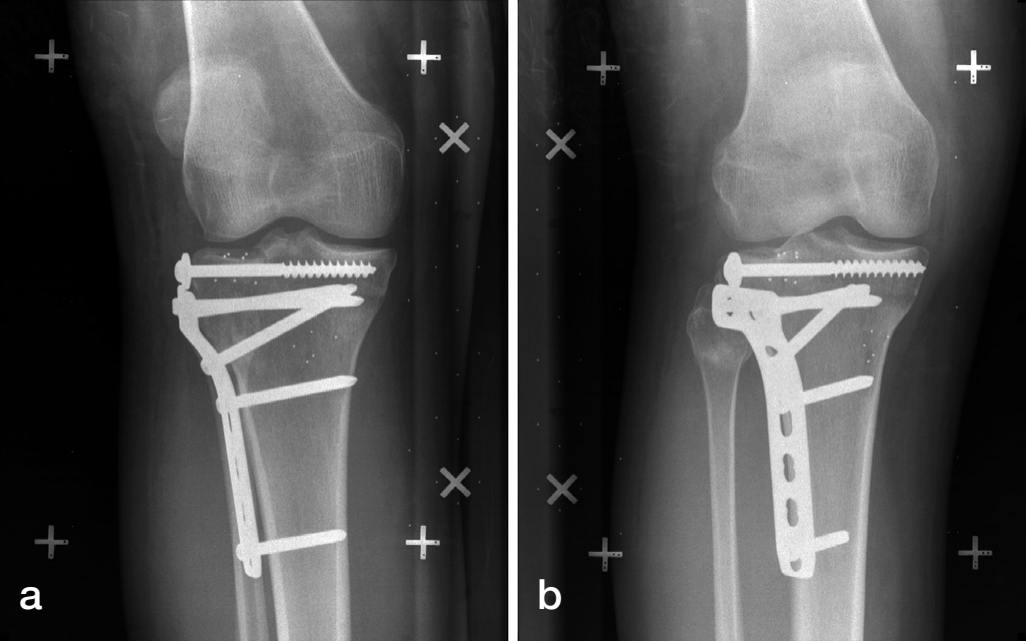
Radiographs of the application of RSA in vivo. Focus 1 (panel a) and focus 2 (panel b).
